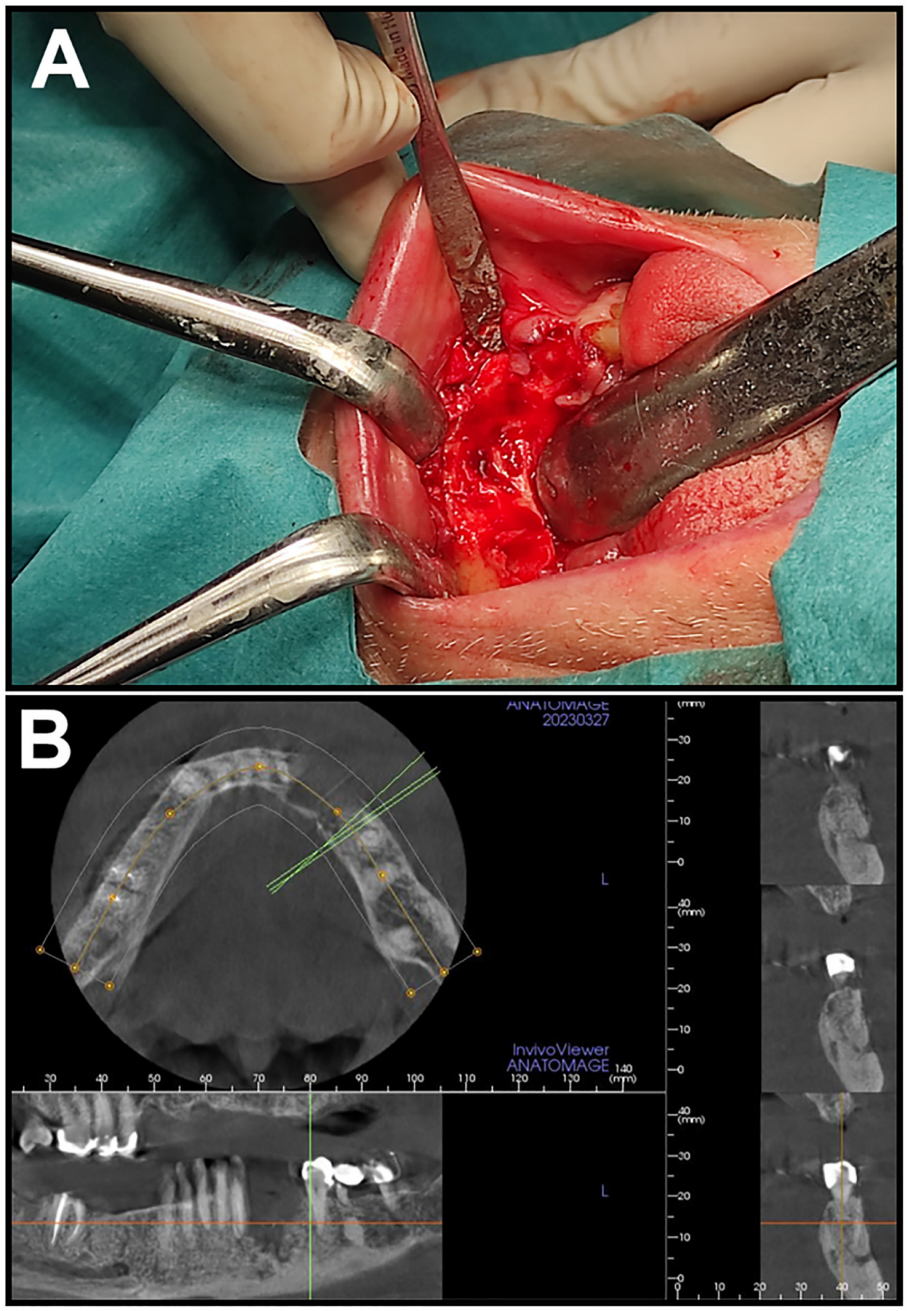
Clinical images corresponding to Case 1 A: Clinical presentation of the patient during surgery; B: Cone-beam CT (CBCT) image of patient before the surgical intervention
